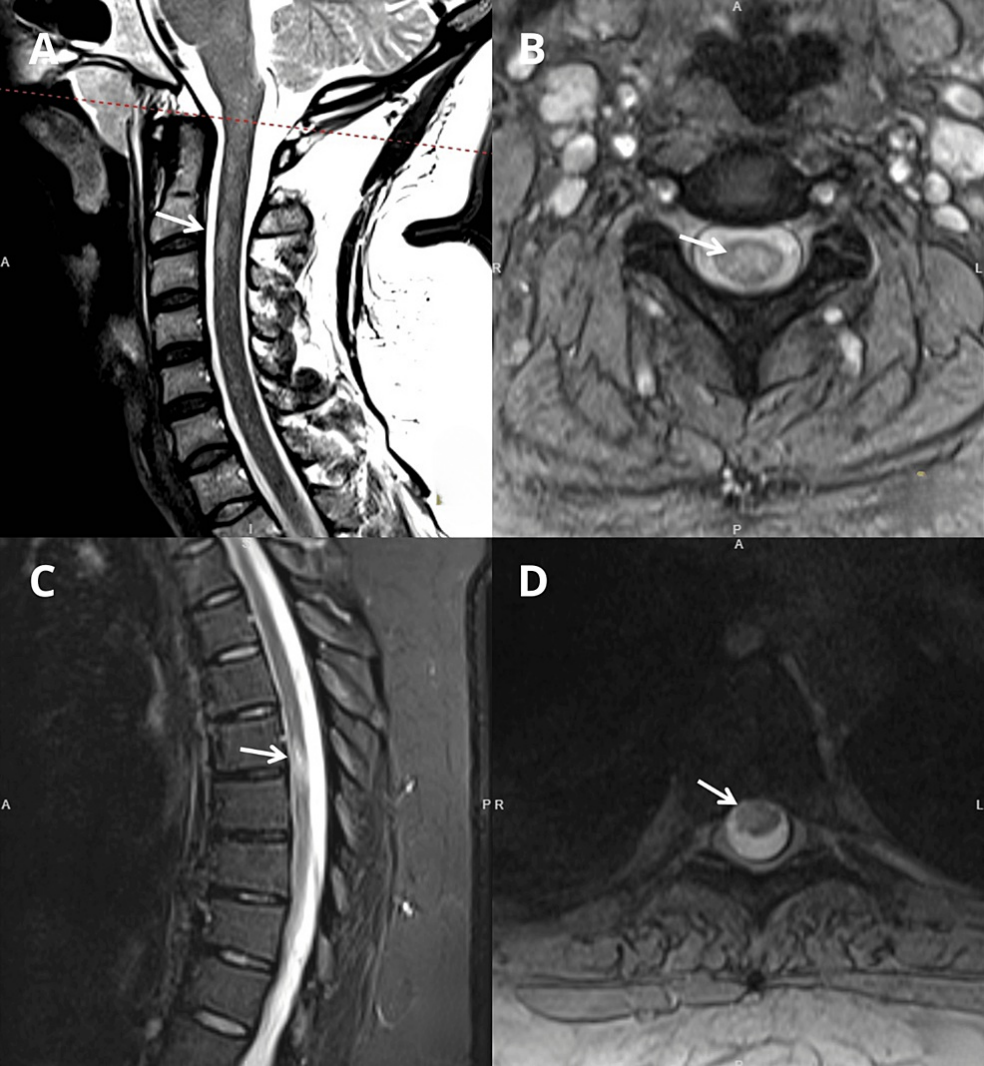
Spinal MRI revealing areas of focal hypersignal in T2 (A) Spinal MRI, sagittal T2, centromedullary hyperintense signal at C2/C3 (arrow). (B) Spinal MRI, axial T2, the same finding described in (A) (arrow). (C) Spinal MRI, sagittal T2 STIR, intramedullary hyperintense signal lateralized to the right at D6/D7 (arrow). (D) Spinal MRI, axial T2, the same finding described in (C) (arrow).
